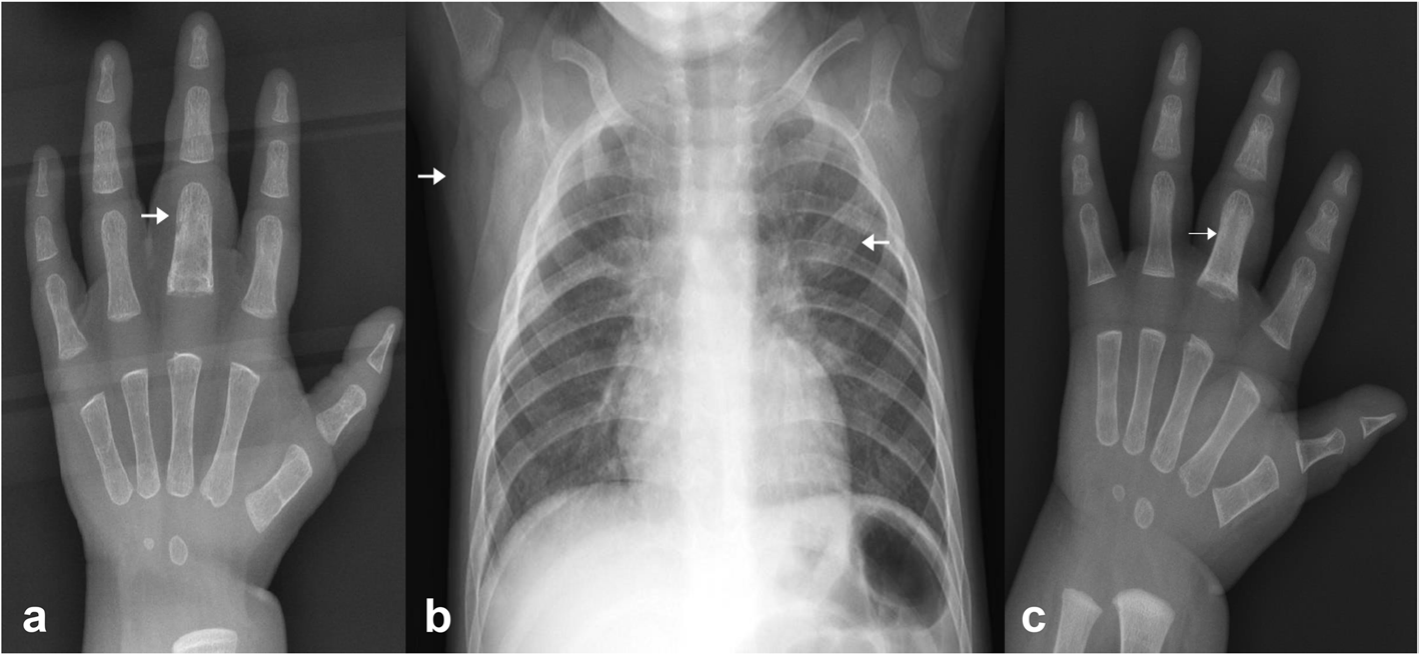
Radiological images of the proband’s half-brother. a AP radiograph of the right hand demonstrates expansion of the proximal 3rd phalanx with multiple ill-defined lucencies consistent with osteiomyelitis. There is associated soft tissue swelling. b AP chest radiograph demonstrates right axillary soft tissue swelling consistent with adenopathy. There is expansion of the 2nd left anterior rib with ill- defined lucencies consistent with osteomyelitis. Lateral x-ray confirmed the absence of mediastinal adenopathy. c AP radiograph done after 4 months demonstrates improvement of the soft tissue swelling, decreased expansion and sclerosis of the 3rd proximal phalanx consistent with healing osteomyelitis
